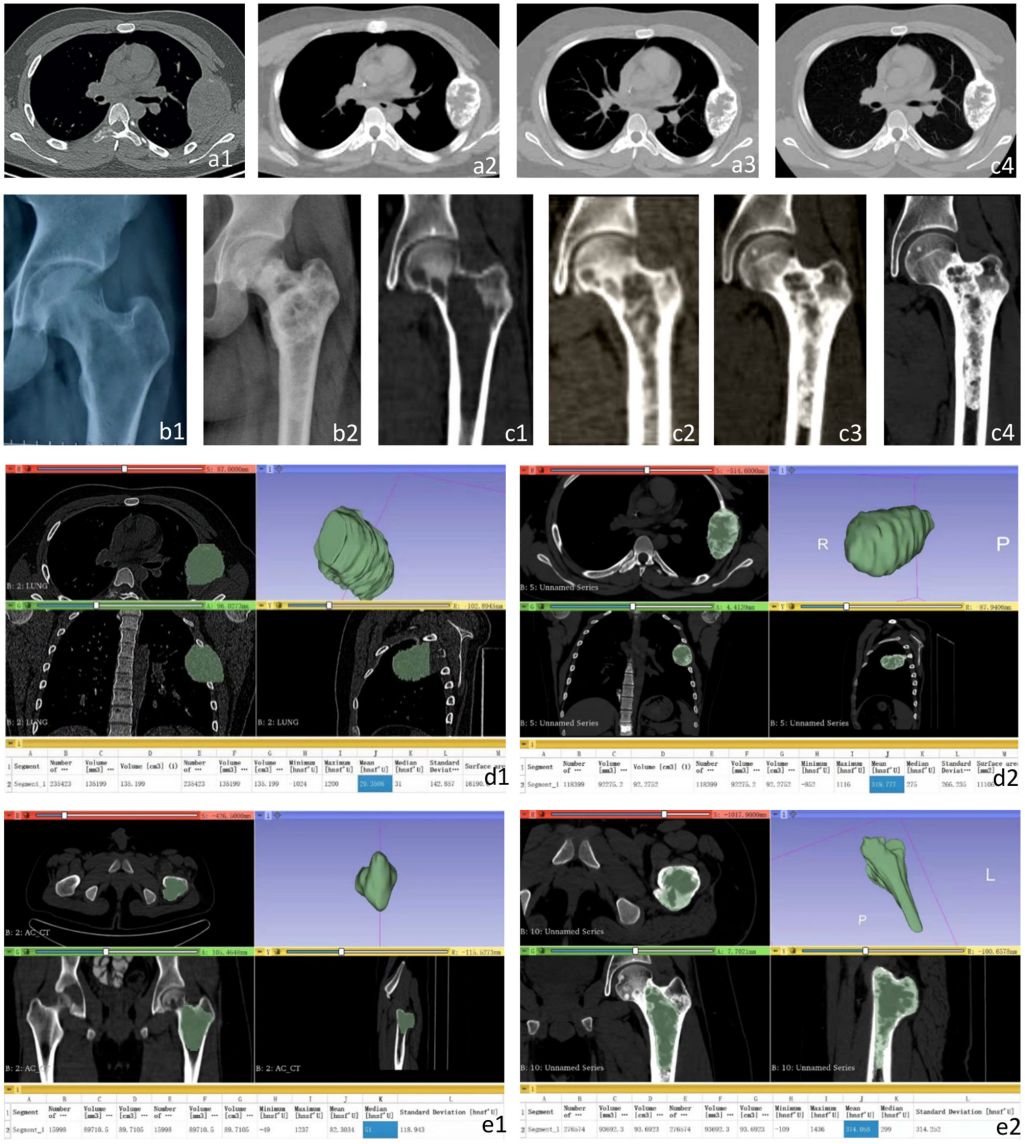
CT and X-ray images of the left fourth rib and proximal left femur before and after treatment. (a1) the left fourth rib showed expansive low-density changes before treatment; (a2–a4) A gradually expanding speckled high-density foci and sclerotic edges was detected in the left fourth rib before the 3^rd^
